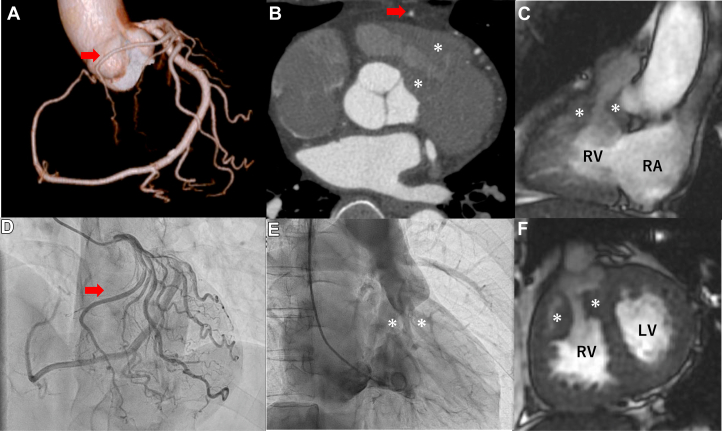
Multimodality Imaging of RVOT Obstruction and Single Coronary Artery (A and B) Cardiac computed tomography showed a single coronary artery arising from the left coronary sinus (Lipton type L1). A branch of the left anterior descending artery courses anteriorly across the right ventricular outflow tract (red arrow). (C and F) Cardiac magnetic resonance demonstrating focal Right Ventricular Outflow Tract (RVOT) narrowing without residual ventricular septal defect (asterisks). (D) Coronary angiography revealing a single coronary artery arising from the left coronary sinus (Lipton type L1). A branch of the left anterior descending artery courses anteriorly across the right ventricular outflow tract (red arrow). (E) Right ventriculography demonstrating severe RVOT obstruction with a marked pressure gradient (asterisks). The asterisks indicate the focal subvalvular narrowing of the right ventricular outflow tract (RVOT). LV = left ventricle; RA = right atrium; RV = right ventricle.
